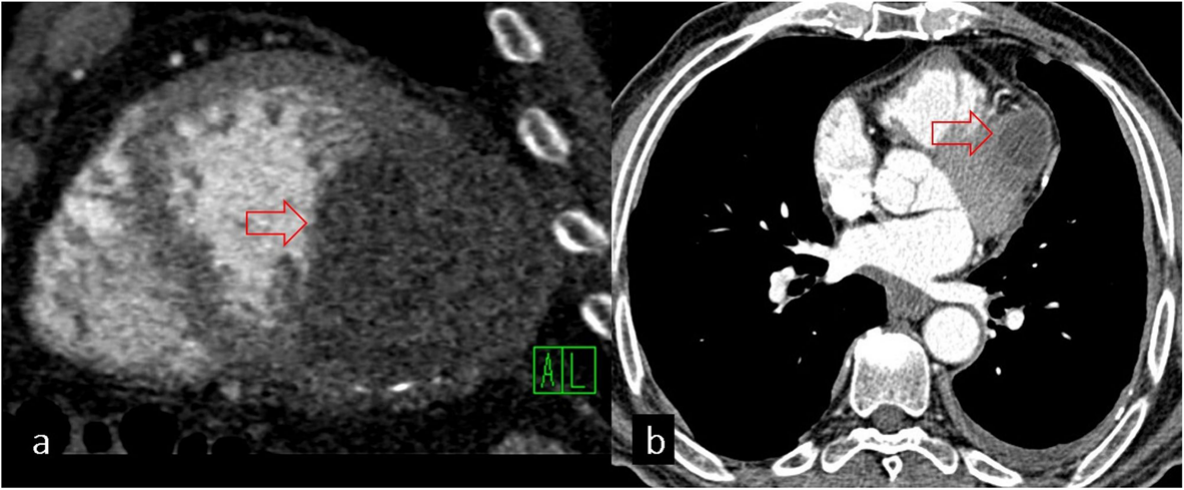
Cardiac involvement of hydatid cyst. a Hydatid cyst with calcification in the left ventricle is seen on the short-axis CT image (red arrow). b Hydatid cyst in the left ventricle is demonstrated in another patient (red arrow)
